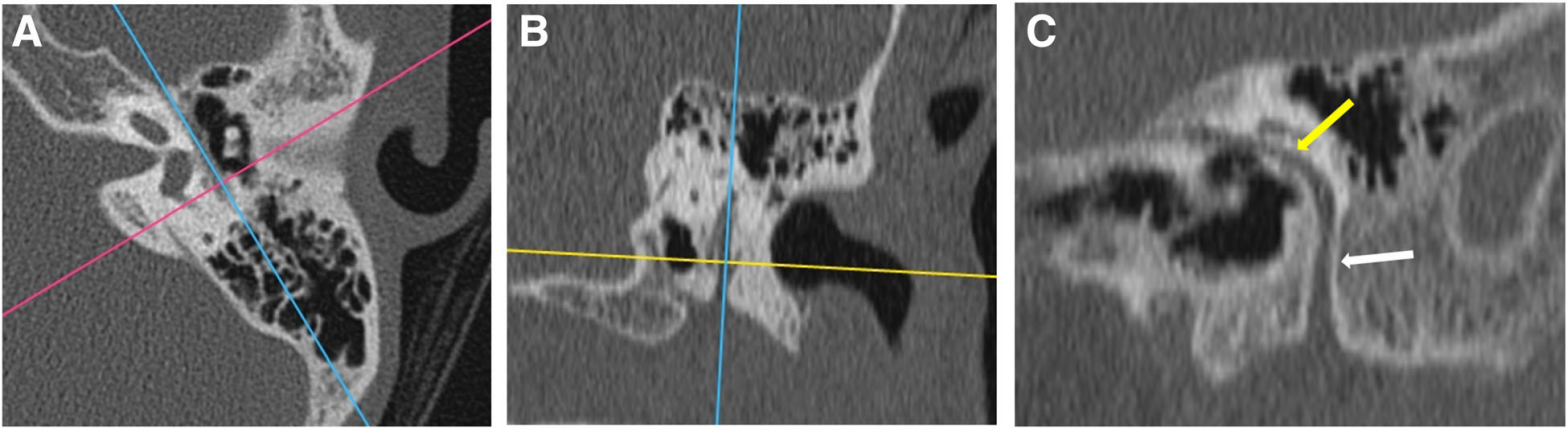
Axial (a) and coronal (b) reference CT images show plane of reconstruction (blue line) to form the double oblique sagittal (c) image showing normal anatomy of tympanic (yellow arrow) and mastoid segments (white arrow) of the facial nerve canal
